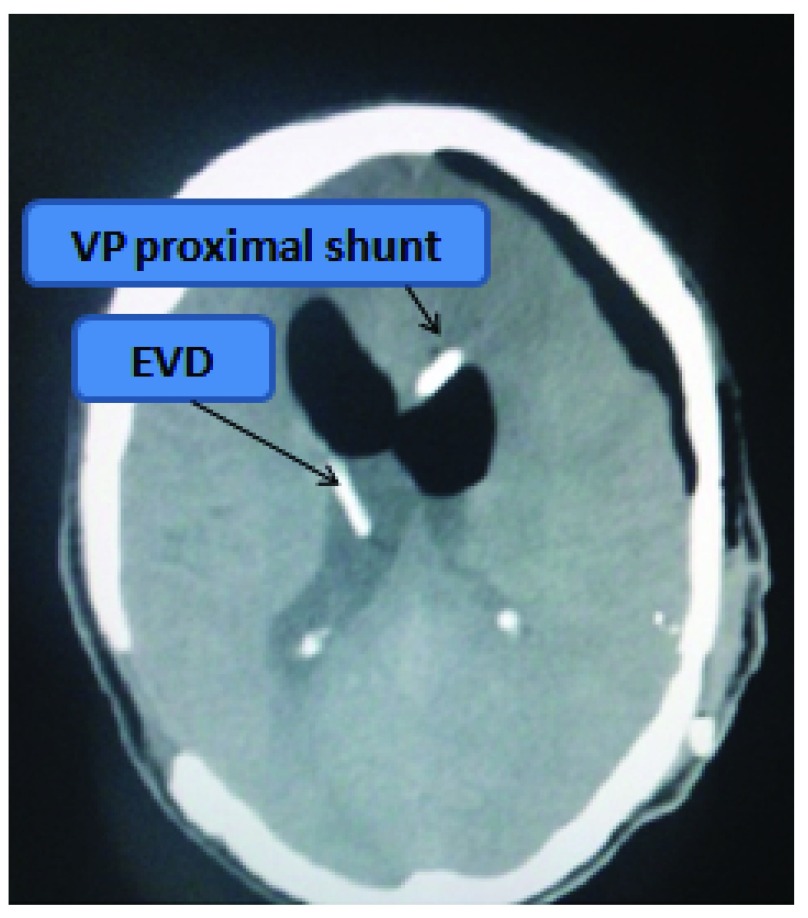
CT image showing location of EVD and VP Shunt proximal end.
